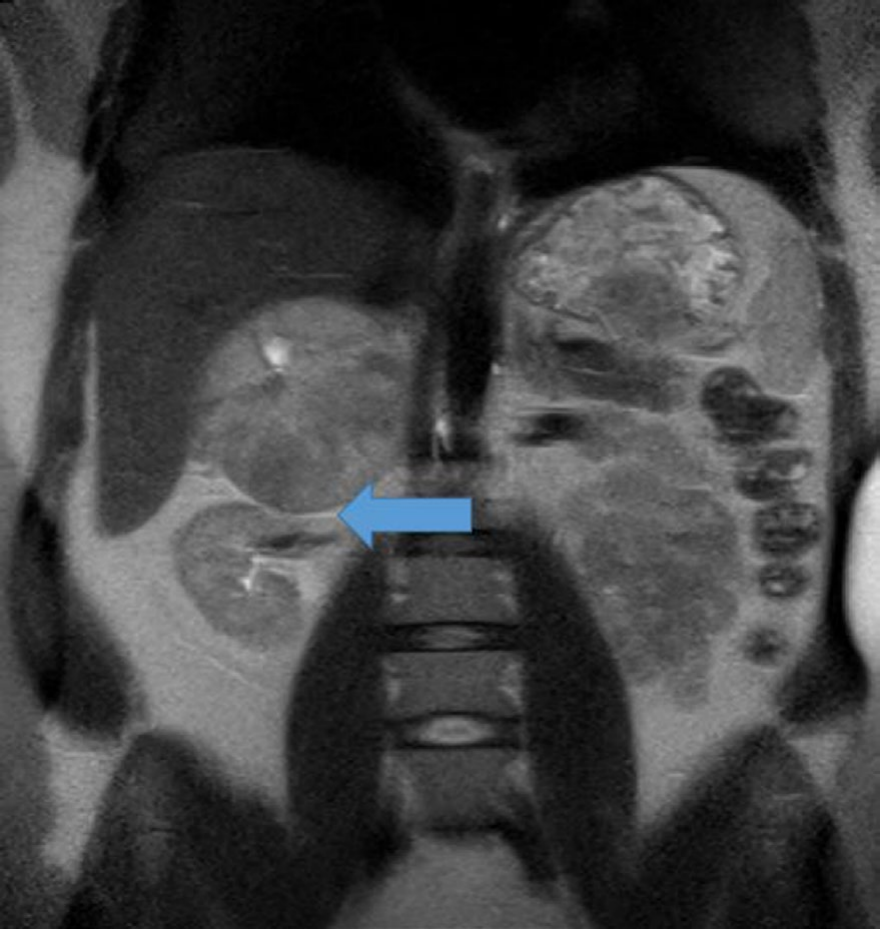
The magnetic resonance imaging shows the pheochromocytoma with a size of about 9.7 × 6.9 cm and good demarcation to the surrounding tissue (above arrow). The inferior vena cava is slightly compressed, as is the kidney (see arrow)
